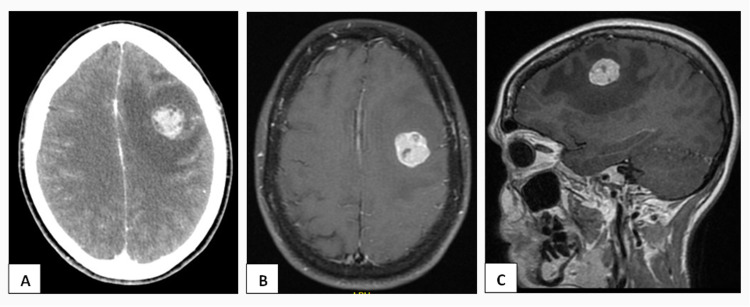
(A) Unique expansive lesion in the left cerebral hemisphere in CT scan (axial acquisition). (B) The same lesion in the brain MRI (axial acquisition) and (C) sagittal acquisition.
